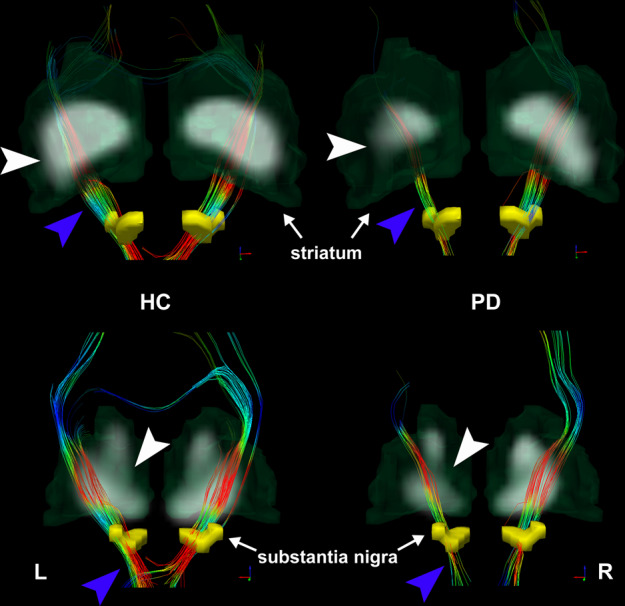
Deterministic tractography images of reconstructed nigrostriatal fibre tracts (projected from substantia nigra to striatum) coregistering with ^18^F-DOPA SUVR parametric images. With different projections, impaired nigrostriatal fibre tracts (blue arrowhead) were distinct in accordance with reduced striatal SUVR (white arrowhead). Colour-encoded orientations: green for anterior–posterior, red for transverse, and blue for superior–inferior directions. Abbreviations: ^18^F-DOPA ^18^F-fluorodopa, SUVR standard uptake value ratio, HC healthy control, PD Parkinson’s disease, L left, R right.
